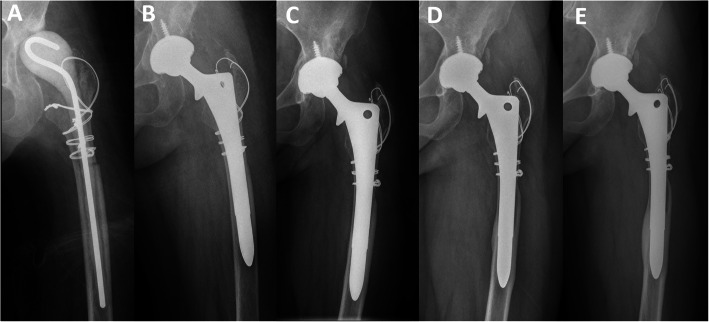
Anteroposterior radiographs of a 48-year-old woman who underwent revision THA for periprosthetic joint infection. a Radiograph prior to stage 2 revision THA showing the antibiotic-loaded spacer in situ. b Radiograph immediately after revision THA using a cementless extensively porous-coated stem without cortical strut allografts. c Postoperative radiograph at 1 year showing loss of cortical density and thickness in the proximal femur, suggesting moderate area and severity of stress shielding. d Postoperative radiograph at 5 years showing severe stress shielding and distal cortical hypertrophy on both the medial and lateral sides. e Postoperative radiograph at 10 years showing more severe bone loss and distal cortical hypertrophy
